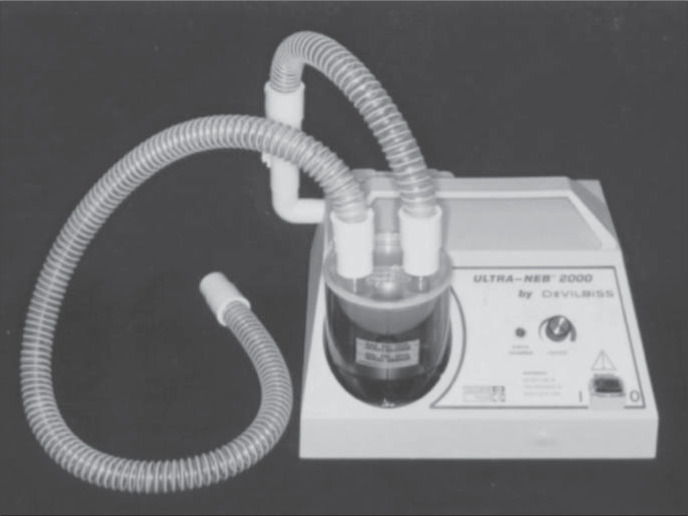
DeVilbiss ultrasonic nebulizer (model Ultra-Neb 2000).
